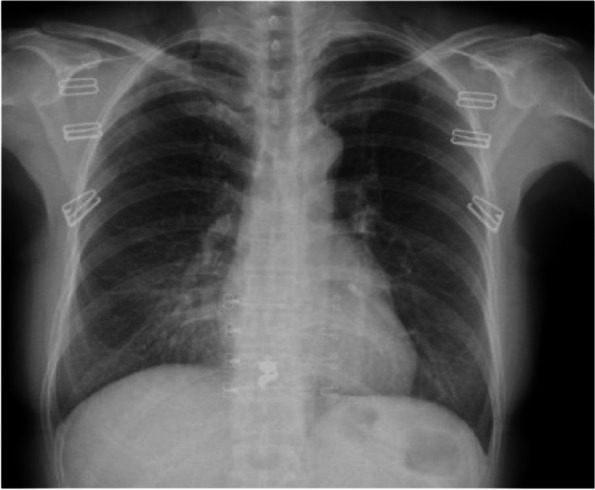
There were no abnormal nodules or space occupying in the lungs on the chest X-ray before first operation
